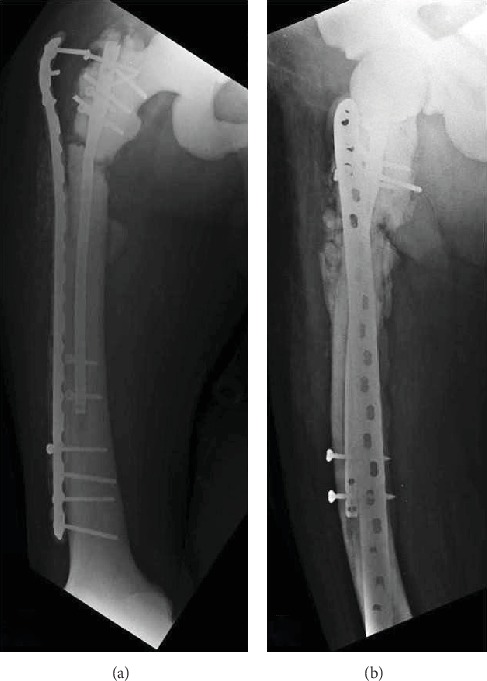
Anteroposterior (a) and lateral (b) radiographs of the femur showing the failure of the first revision configuration.
